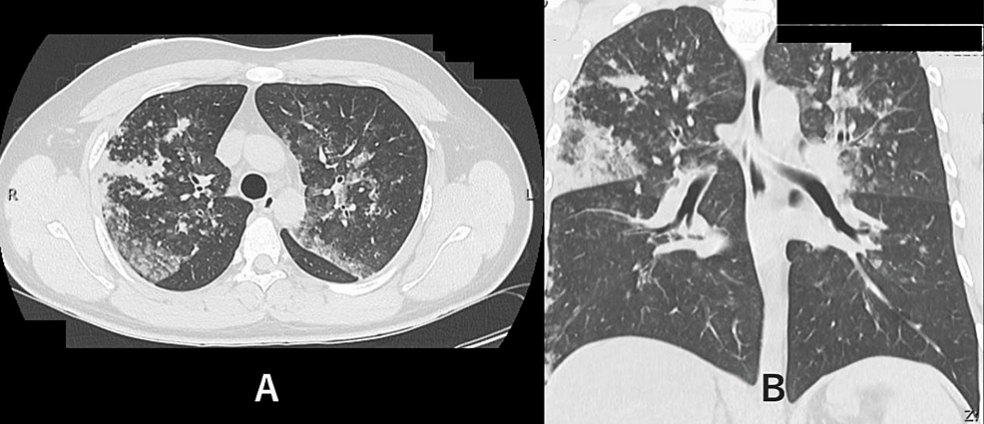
Chest computed tomography at the time of arrival A: axial view. B: coronal view.
